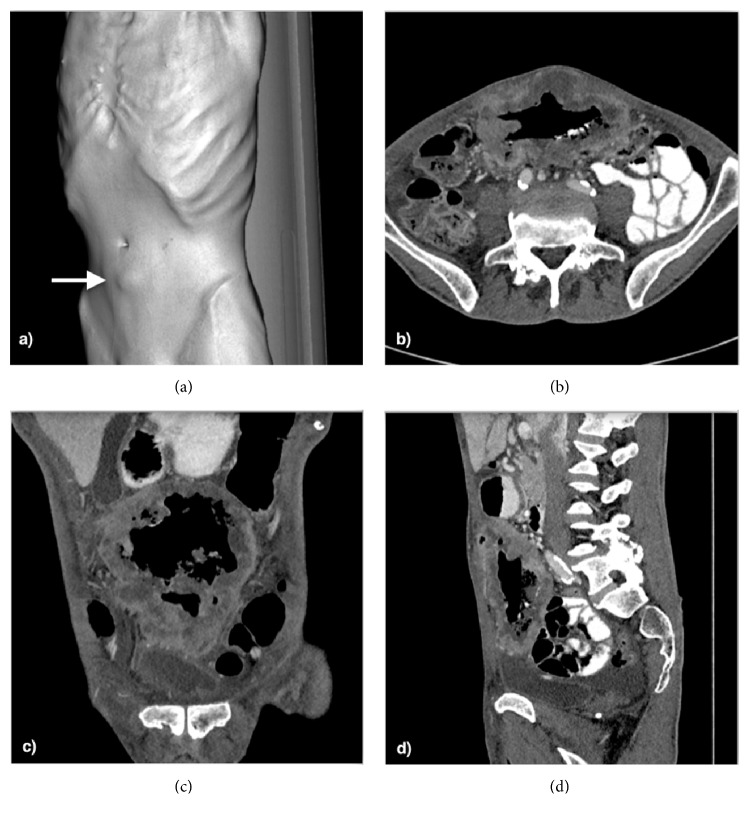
(a) 3D CT reconstruction showing an emaciated patient with a visible infraumbilical mass (arrow). Axial (b), coronal (c), and sagittal (d) contrast-enhanced CT images depicting a large and heterogeneous median abdominal mass, extending from the anterosuperior aspect of the bladder toward the umbilicus.
